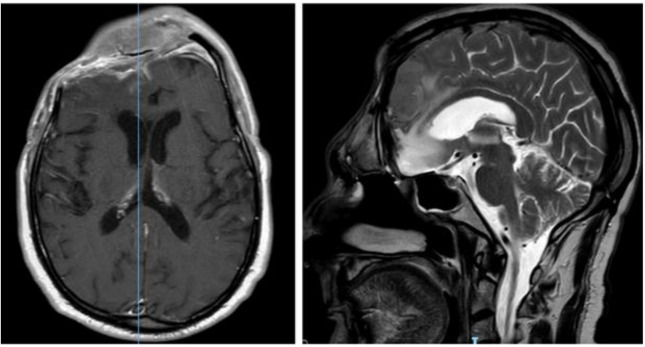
Post-operative brain MRI after evacuation of the epidural empyema, cranial bone flap removal and reconstruction by means of anterolateral thigh flap.
